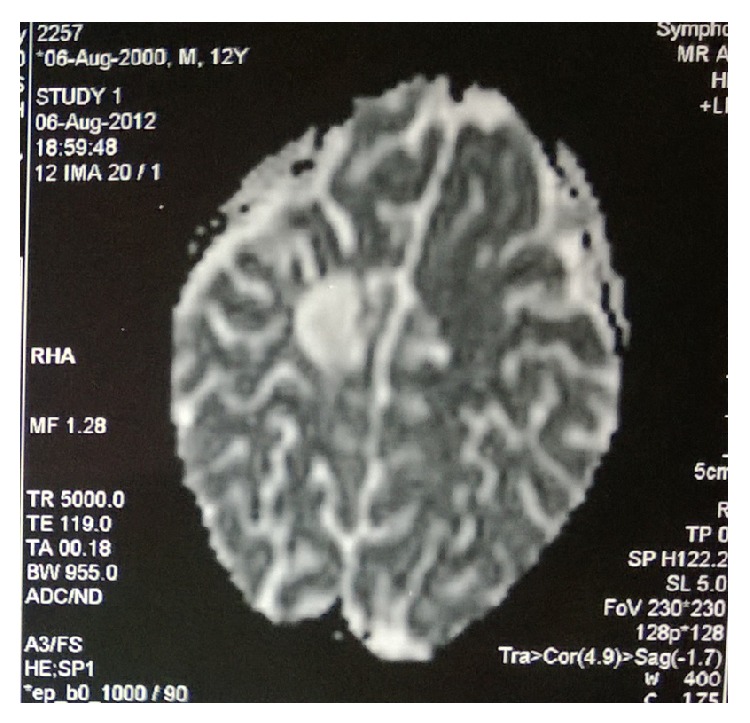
CT scan residual tumor 2012.
